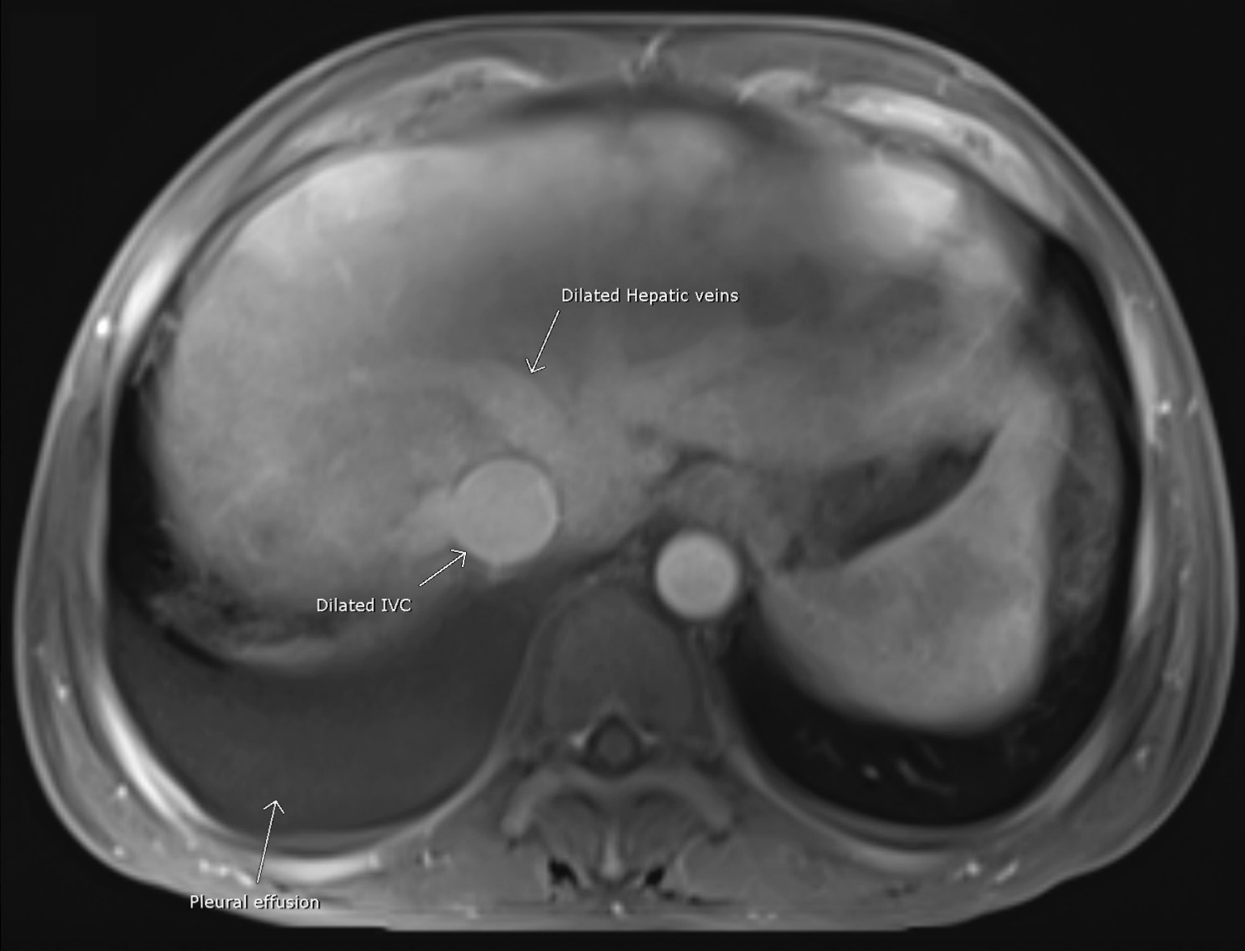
MRI abdomen with intravenous contrast showing a dilated inferior vena cava (IVC) and prominent hepatic veins in addition to right‐sided pleural effusion.
